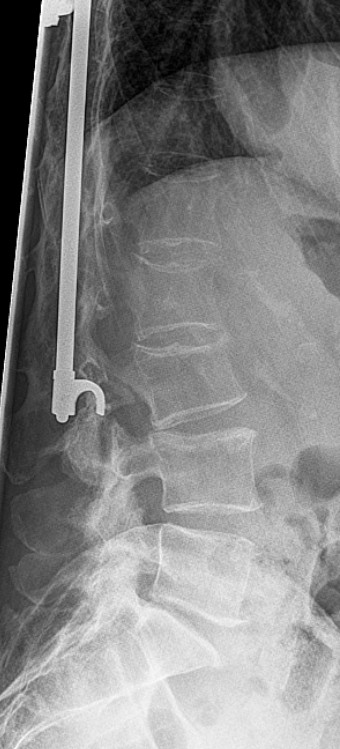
X-ray of the lumbar spine in lateral projection showing the fractured pedicle
